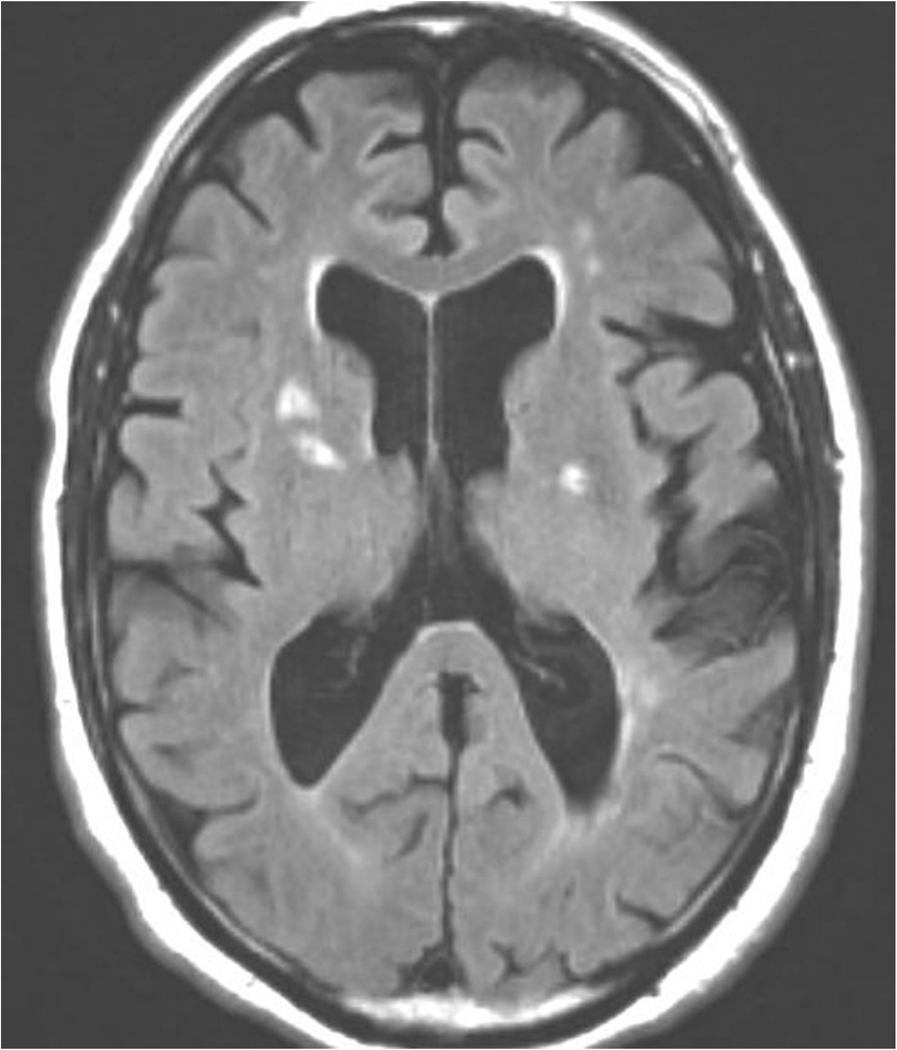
Initial MRI FLAIR sequence. MRI FLAIR sequence showing several bilateral areas of discreet cerebral ischemia. The diffusion weighted images were negative, suggesting an absence of acute cerebral infarction related to the continued limb-shaking TIAs
